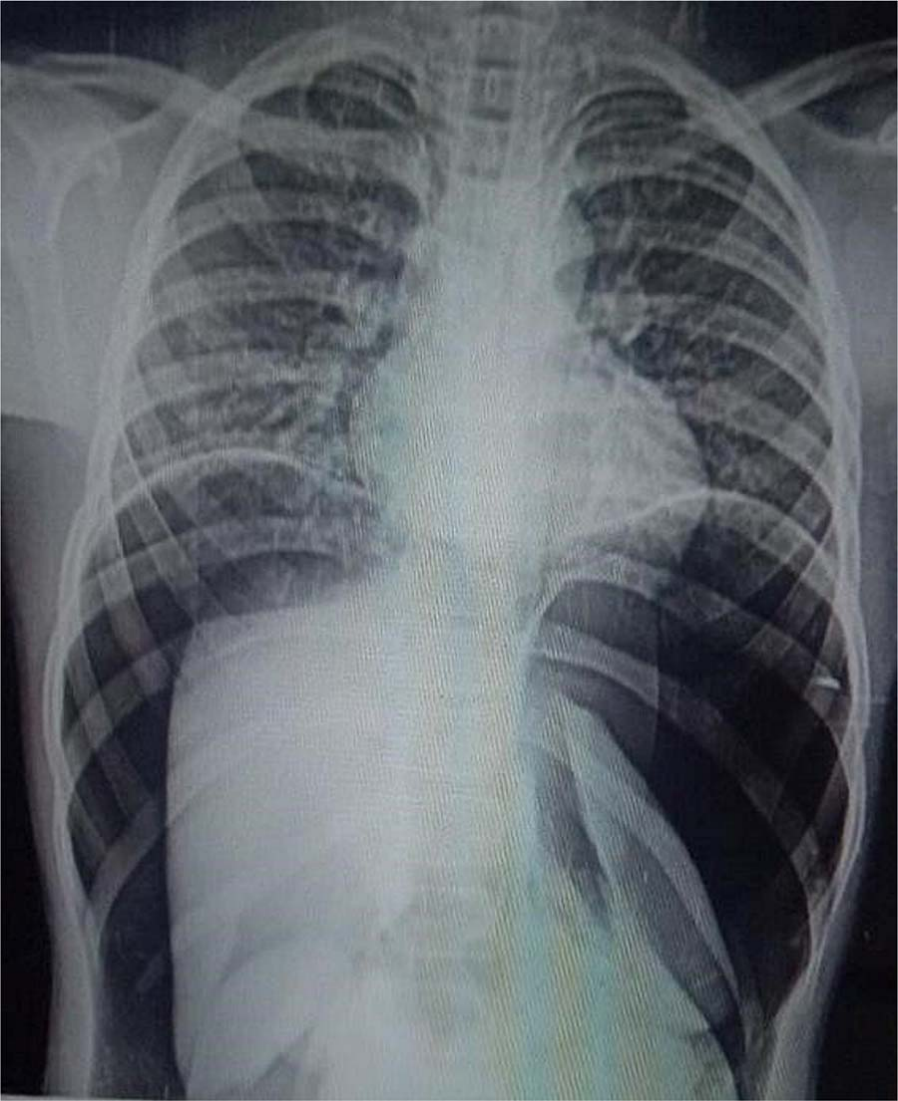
Chest X-rays with pneumoperitoneum.
